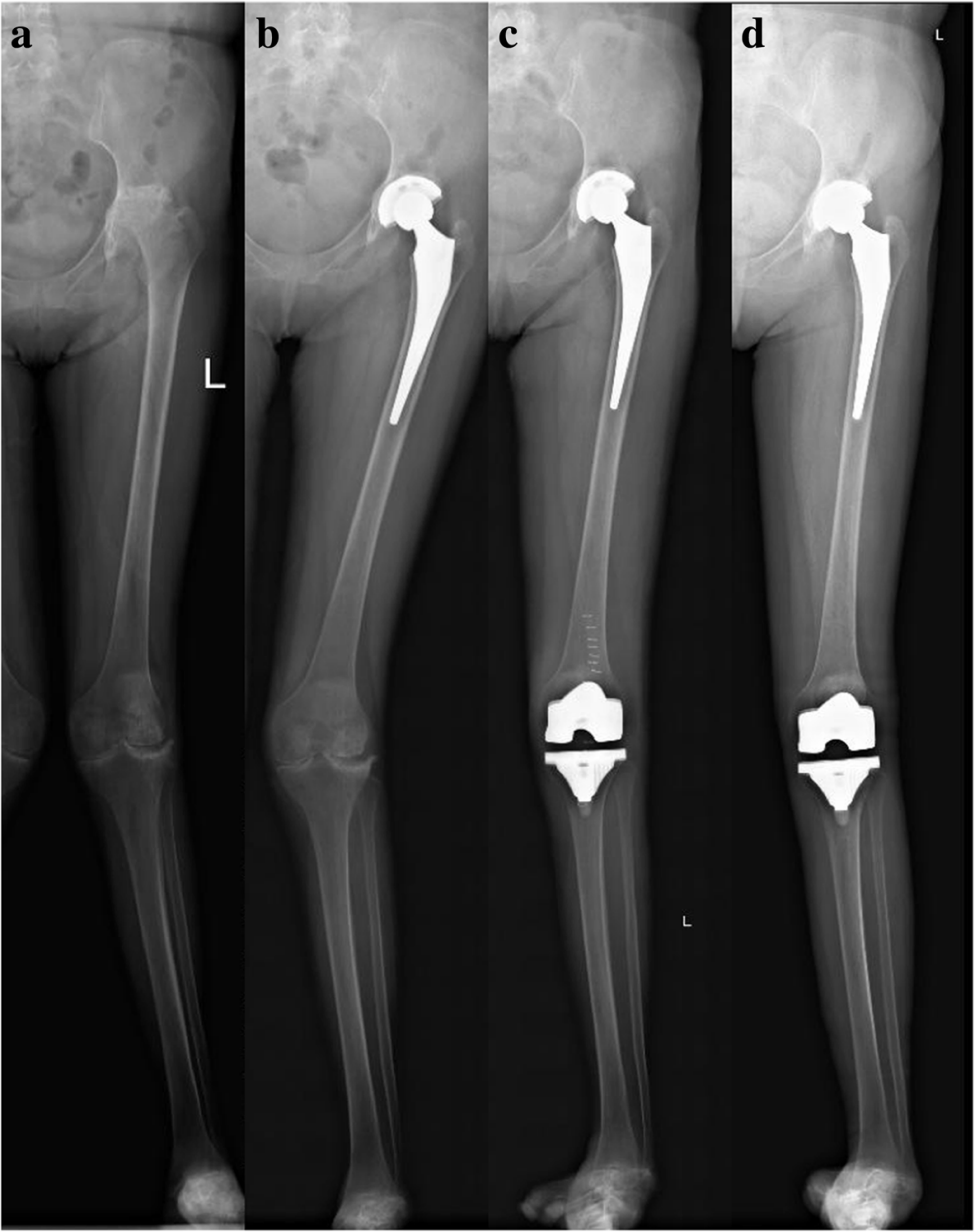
A 43-year-old female with rheumatoid arthritis receiving TKA subsequent to ipsilateral THA. X-ray radiography at preoperative (a) and radiography at 3-month follow-up after THA (b). Seven months after THA, the patient received ipsilateral TKA. The postoperative radiograph of the lower extremity (c) showed stable implant fixation. Postoperative radiographic image (d) at 7-year follow-up demonstrated that the acetabular, femoral, and tibial components were stable
